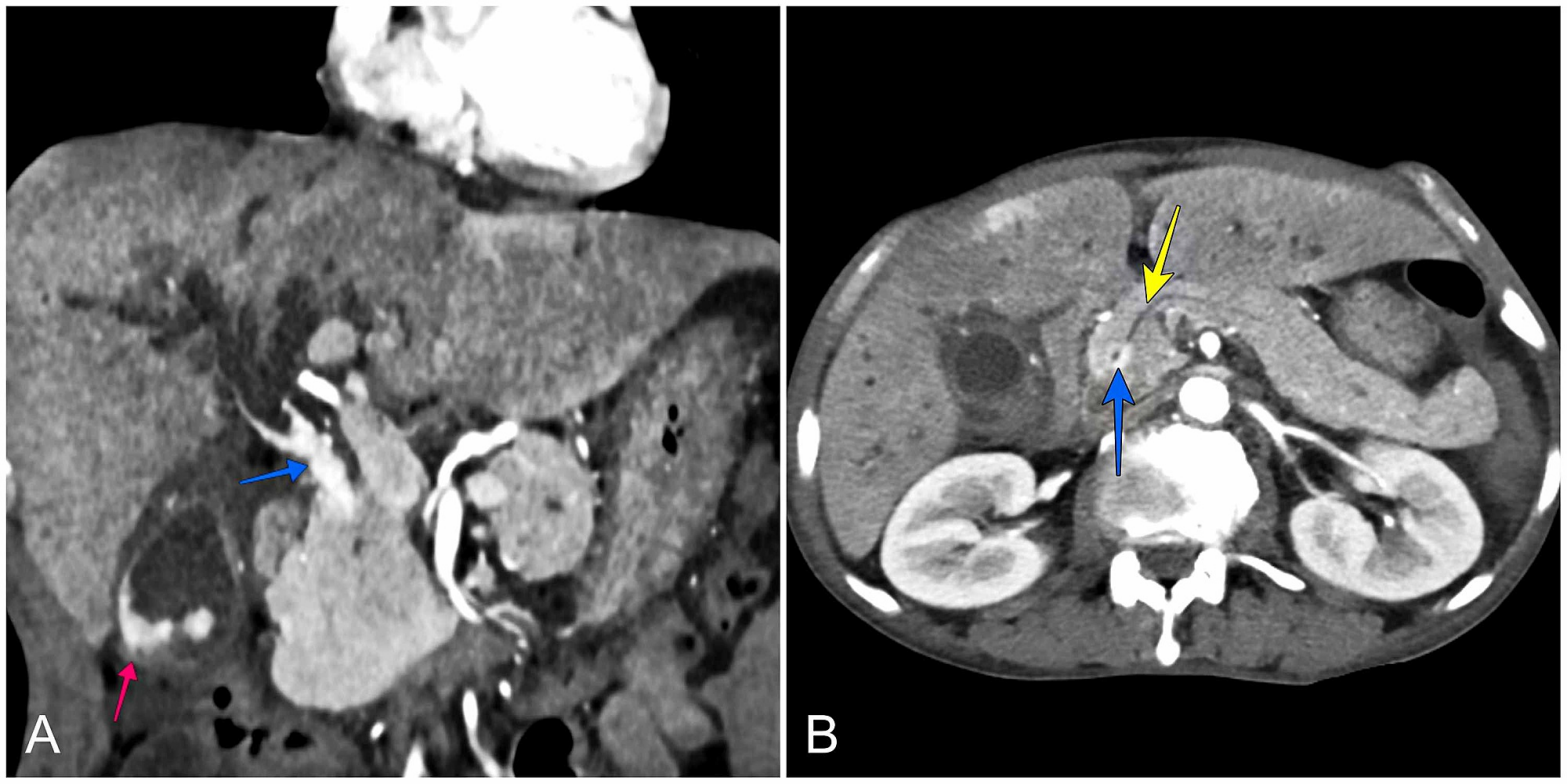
CECT images - arterial phase A: coronal section showing hyperenhancing growth in the gallbladder (red arrow) and the bile duct (blue arrow); B: axial section showing the pancreatic duct (yellow arrow) joining the common bile duct with a circumferential enhancing growth (blue arrow) in the head of the pancreas (outside the duodenal wall) CECT: contrast-enhanced computed tomography
